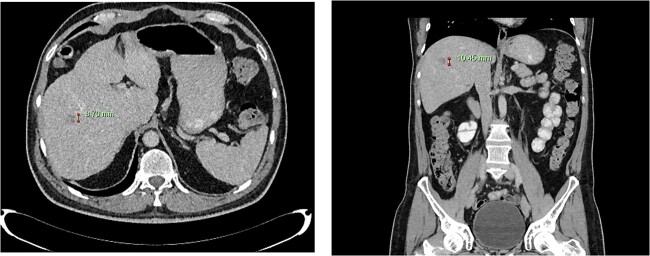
Computed tomography dated 12 March 2013 of the abdomen and pelvis measuring 8.70 mm (anterior–posterior) lesion at liver segment 5/6 in axial (left) and coronal (right) view.
